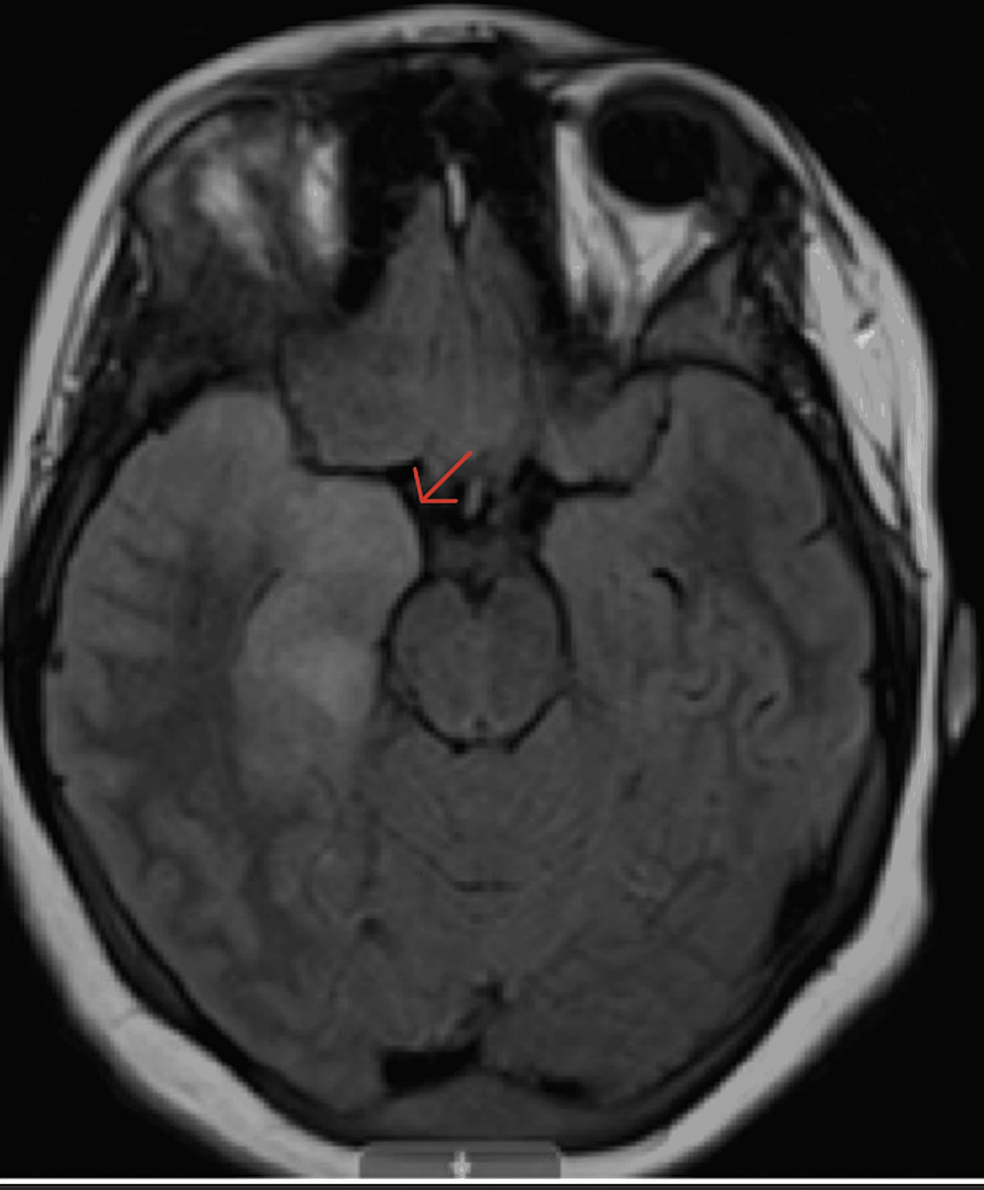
MRI of the brain without contrast in a T1-weighted axial view The axial view shows an enlarged right mesial and lateral temporal lobes with abnormal signal intensity (red arrow).
